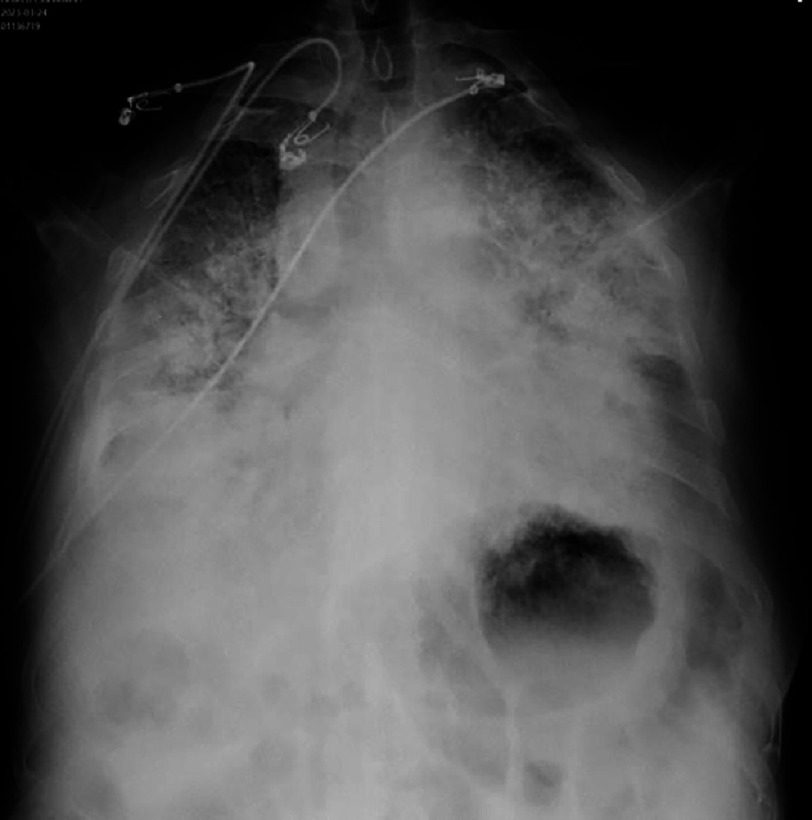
Follow-up chest X-ray, posteroanterior view, showing almost a white lung (Patient #1).
